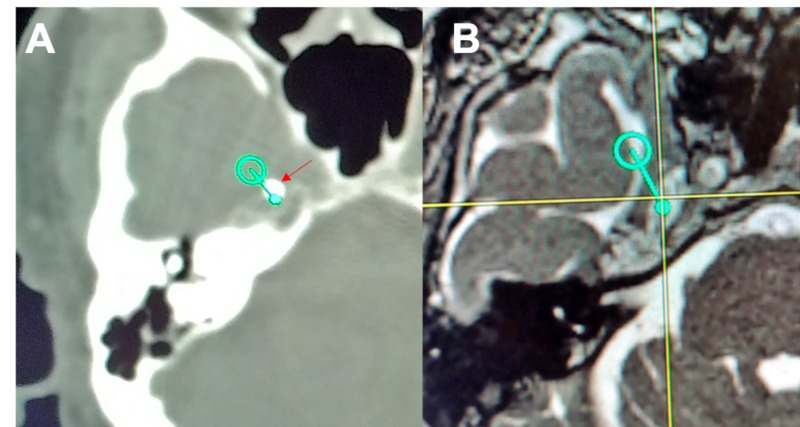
Intraoperative confirmation of adequate needle placement (A) Post-needle placement intraoperative CT showing position of needle tip (arrow) as seen on the stereotactic planning computer. The open green circle indicates the relative position of the foramen ovale (on a different slice). The thin green line represents the trajectory from the foramen ovale to the target in Meckel's cave. The solid green circle indicates the intersection of the trajectory on this CT slice. It can be seen that the needle tip is on the trajectory line between the foramen ovale and the intended target, just short of the planned target. (B) This is a derived image from the stereotactic planning computer showing a preoperative T2 MRI scan slice fused with the intraoperative CT slice just shown. The stereotactic coordinates of the needle tip are transposed as cross-hair to this MRI image of Meckel's cave, with planned trajectory shown in green. Deviation of around 2 mm between the needle tip coordinates and the target in Meckel's cave was noted. The needle tip is clearly in Meckel's cave, and there was good CSF return at this location. No further adjustments to the needle were made, and glycerol was injected with excellent patient outcome.
